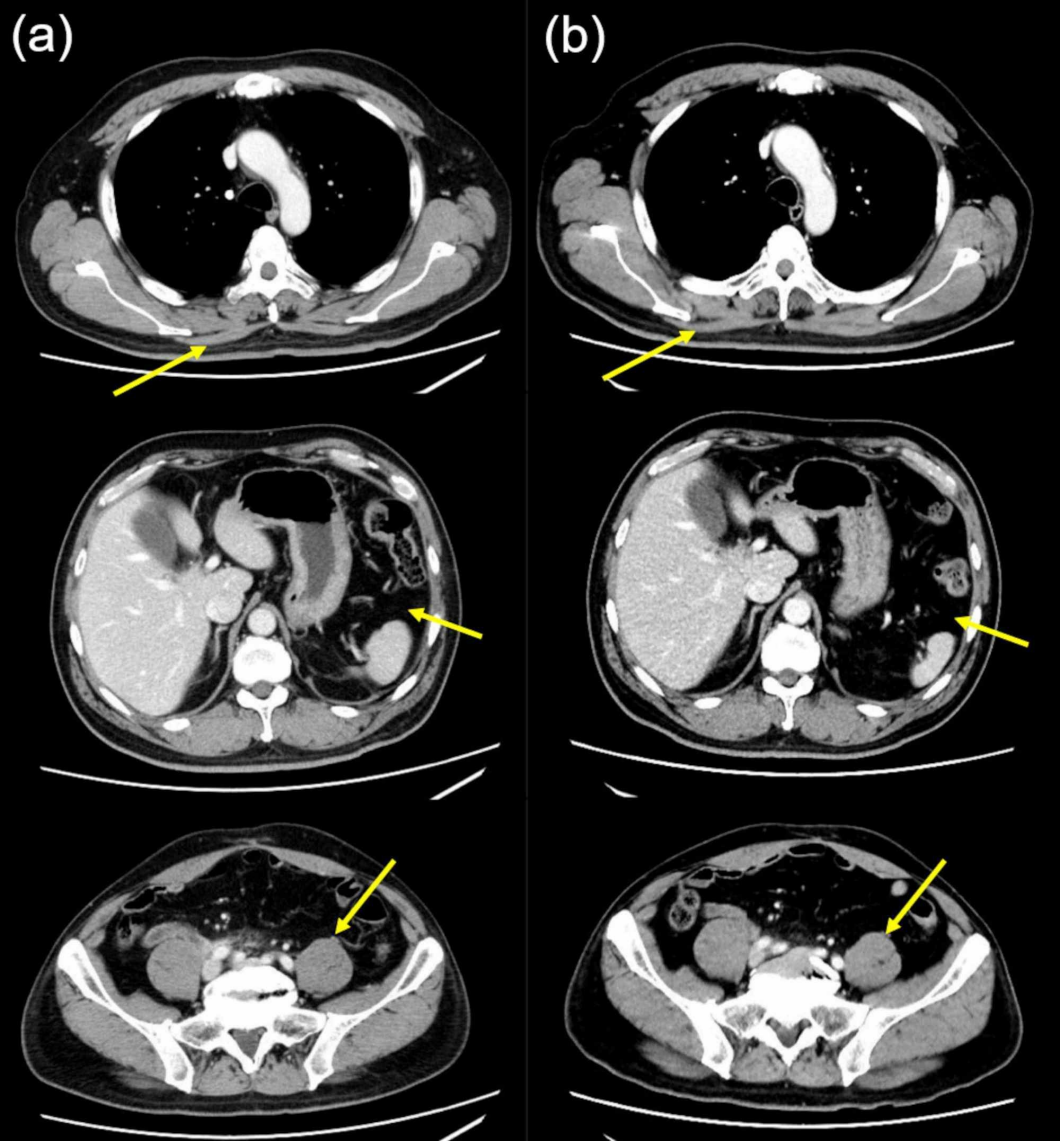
CT scan of a 55-year-old man (body weight, 80.0 kg; body mass index, 26.1) with postoperative bladder cancer (a) A series of axial images reconstructed by AIDR 3D in January 2017 after injecting a contrast medium (550 mg iodine/kg) and scanning the equilibrium phase of the chest-pelvic computed tomography (CT) at 120 kV. (b) A series of axial images reconstructed by FIRST in May 2017 after injecting a contrast medium (330 mg iodine/kg) and scanning the equilibrium phase of the chest-pelvic CT at 80 kV. (a and b) Top image shows the chest, middle image shows the superior abdomen, and bottom image shows the pelvis. The visual score was good for all (a) and (b) from all the observers in contrast enhancement, image noise, artifacts, and overall image quality. However, sharpness in (b) was inferior to all observers in comparison to (a). Areas with low contrast, such as the muscles and fats indicated by the arrows, were rated particularly poor sharpness. The effective dose was reduced by 34.9% (24.9 mSv to 16.2 mSv) by scanning with 80-kV FIRST as opposed to 120-kV AIDR 3D, and the size-specific dose estimate was reduced by 33.6% (25.0 mGy to 16.6 mGy). CT, computed tomography; AIDR 3D, adaptive iterative dose reduction 3D; FIRST, forward-projected model-based iterative reconstruction solution
